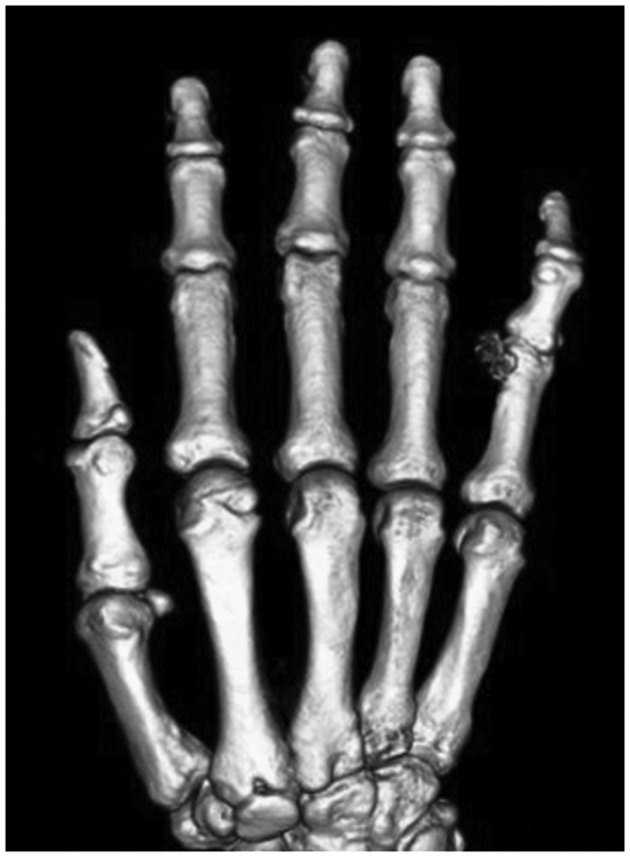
Three-dimensional computed tomography image demonstrating the presence of the lesion.
